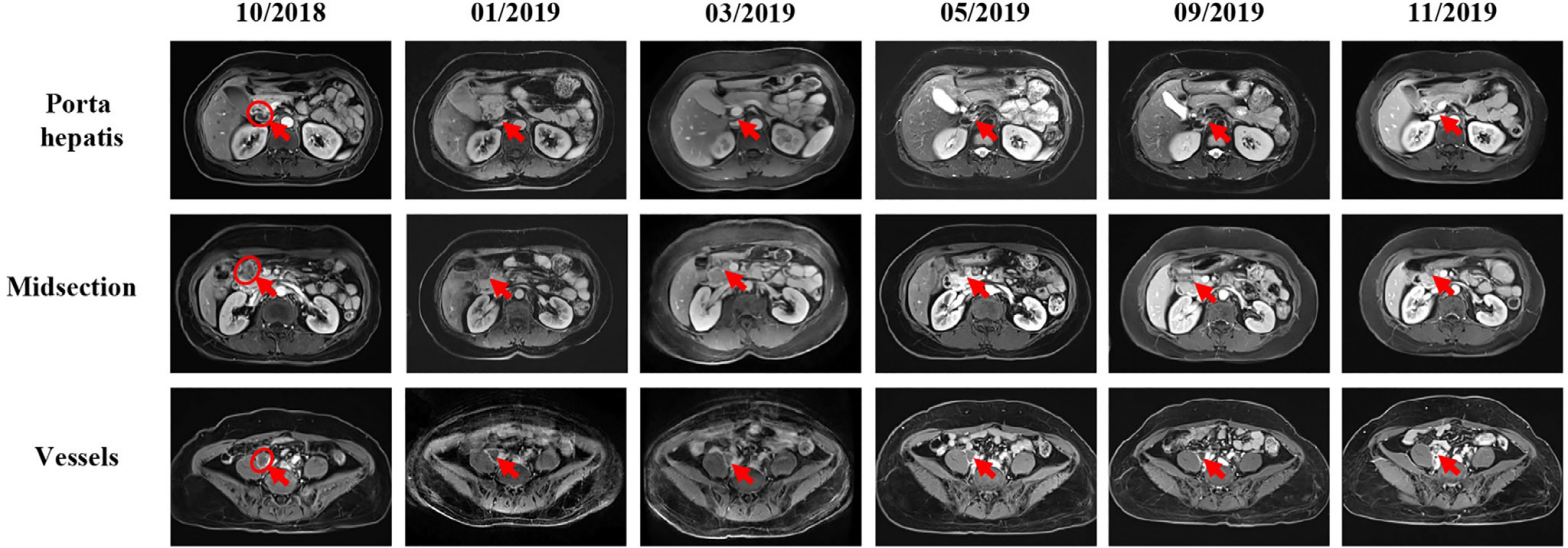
MRI scans show the chronological response of the patient following a series of treatment. The circle represents the area of the target lesion, and arrows indicate the location of the lesions (lymph nodes of porta hepatis, midsection, and vessels). After treatment with FORFIRI and bevacizumab combined, enlarged tumor was observed in October 2018 (lymph nodes of porta hepatis: 23×11mm, midsection: 24×20mm, vessels: 24×18mm). From the change of immunotherapy to November 2019, MRI showed that the tumor was gradually shrinking (The size of hilar hepatis and epigastric mass is not obvious, and the size of vessel is about 16×15mm.).
